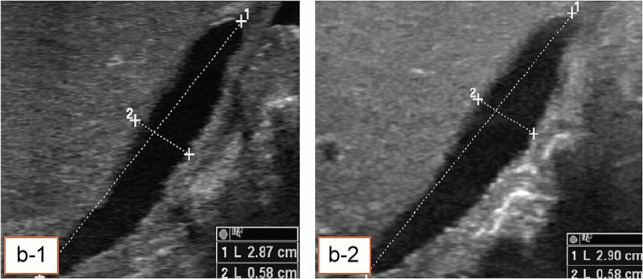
Ultrasound image of an atretic GB. b-1, length (1) and width (2) after 10 hours of fasting. b-2, the same GB after drinking a bottle of milk. No difference in measurements was observed, suggesting GB abnormality—biliary atresia.
